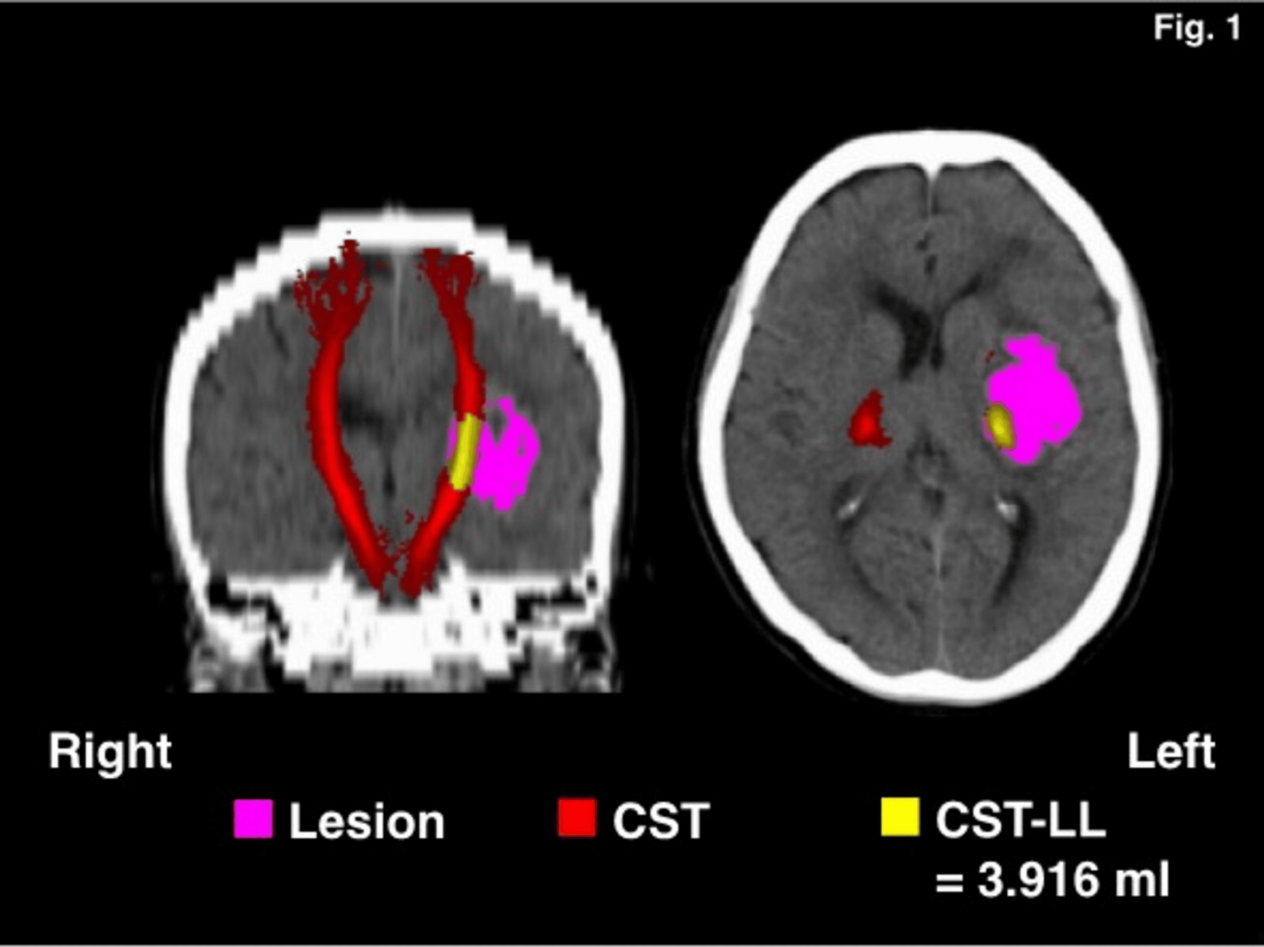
Representative computed tomography images of the brain after spatial registration to a standard brain. CST, corticospinal tract; LL, lesion load.
